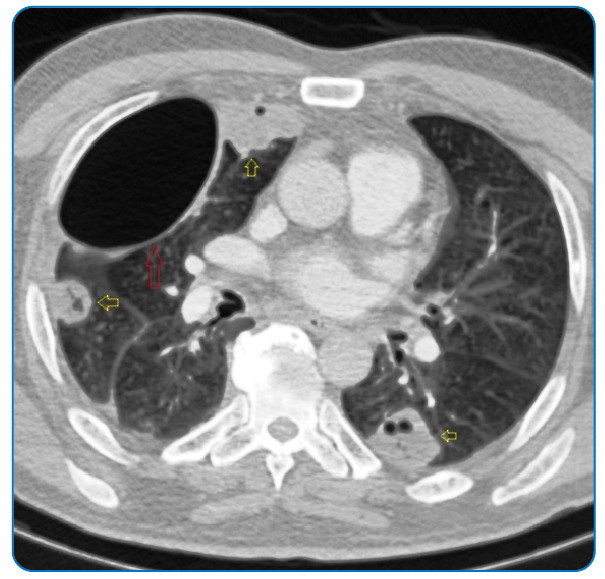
Axial chest CT shows cavitary subpleural nodules (open yellow arrows) and residual pneumothorax (open red arrow).
